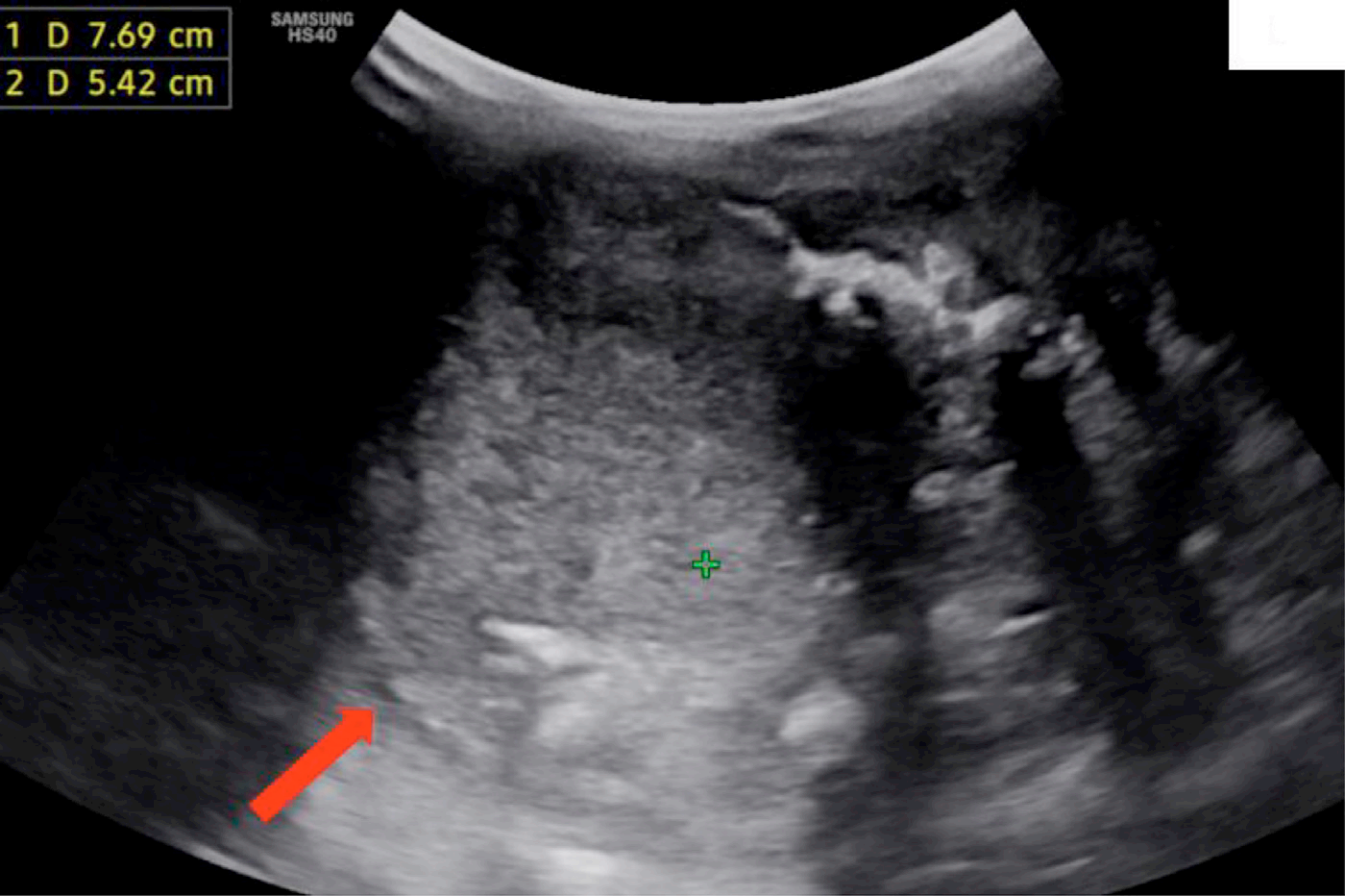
Cervical ultrasound, axial view: A collection involving the left thyroid lobe with echogenic content containing calcifications, generating posterior acoustic shadowing (red arrow).
